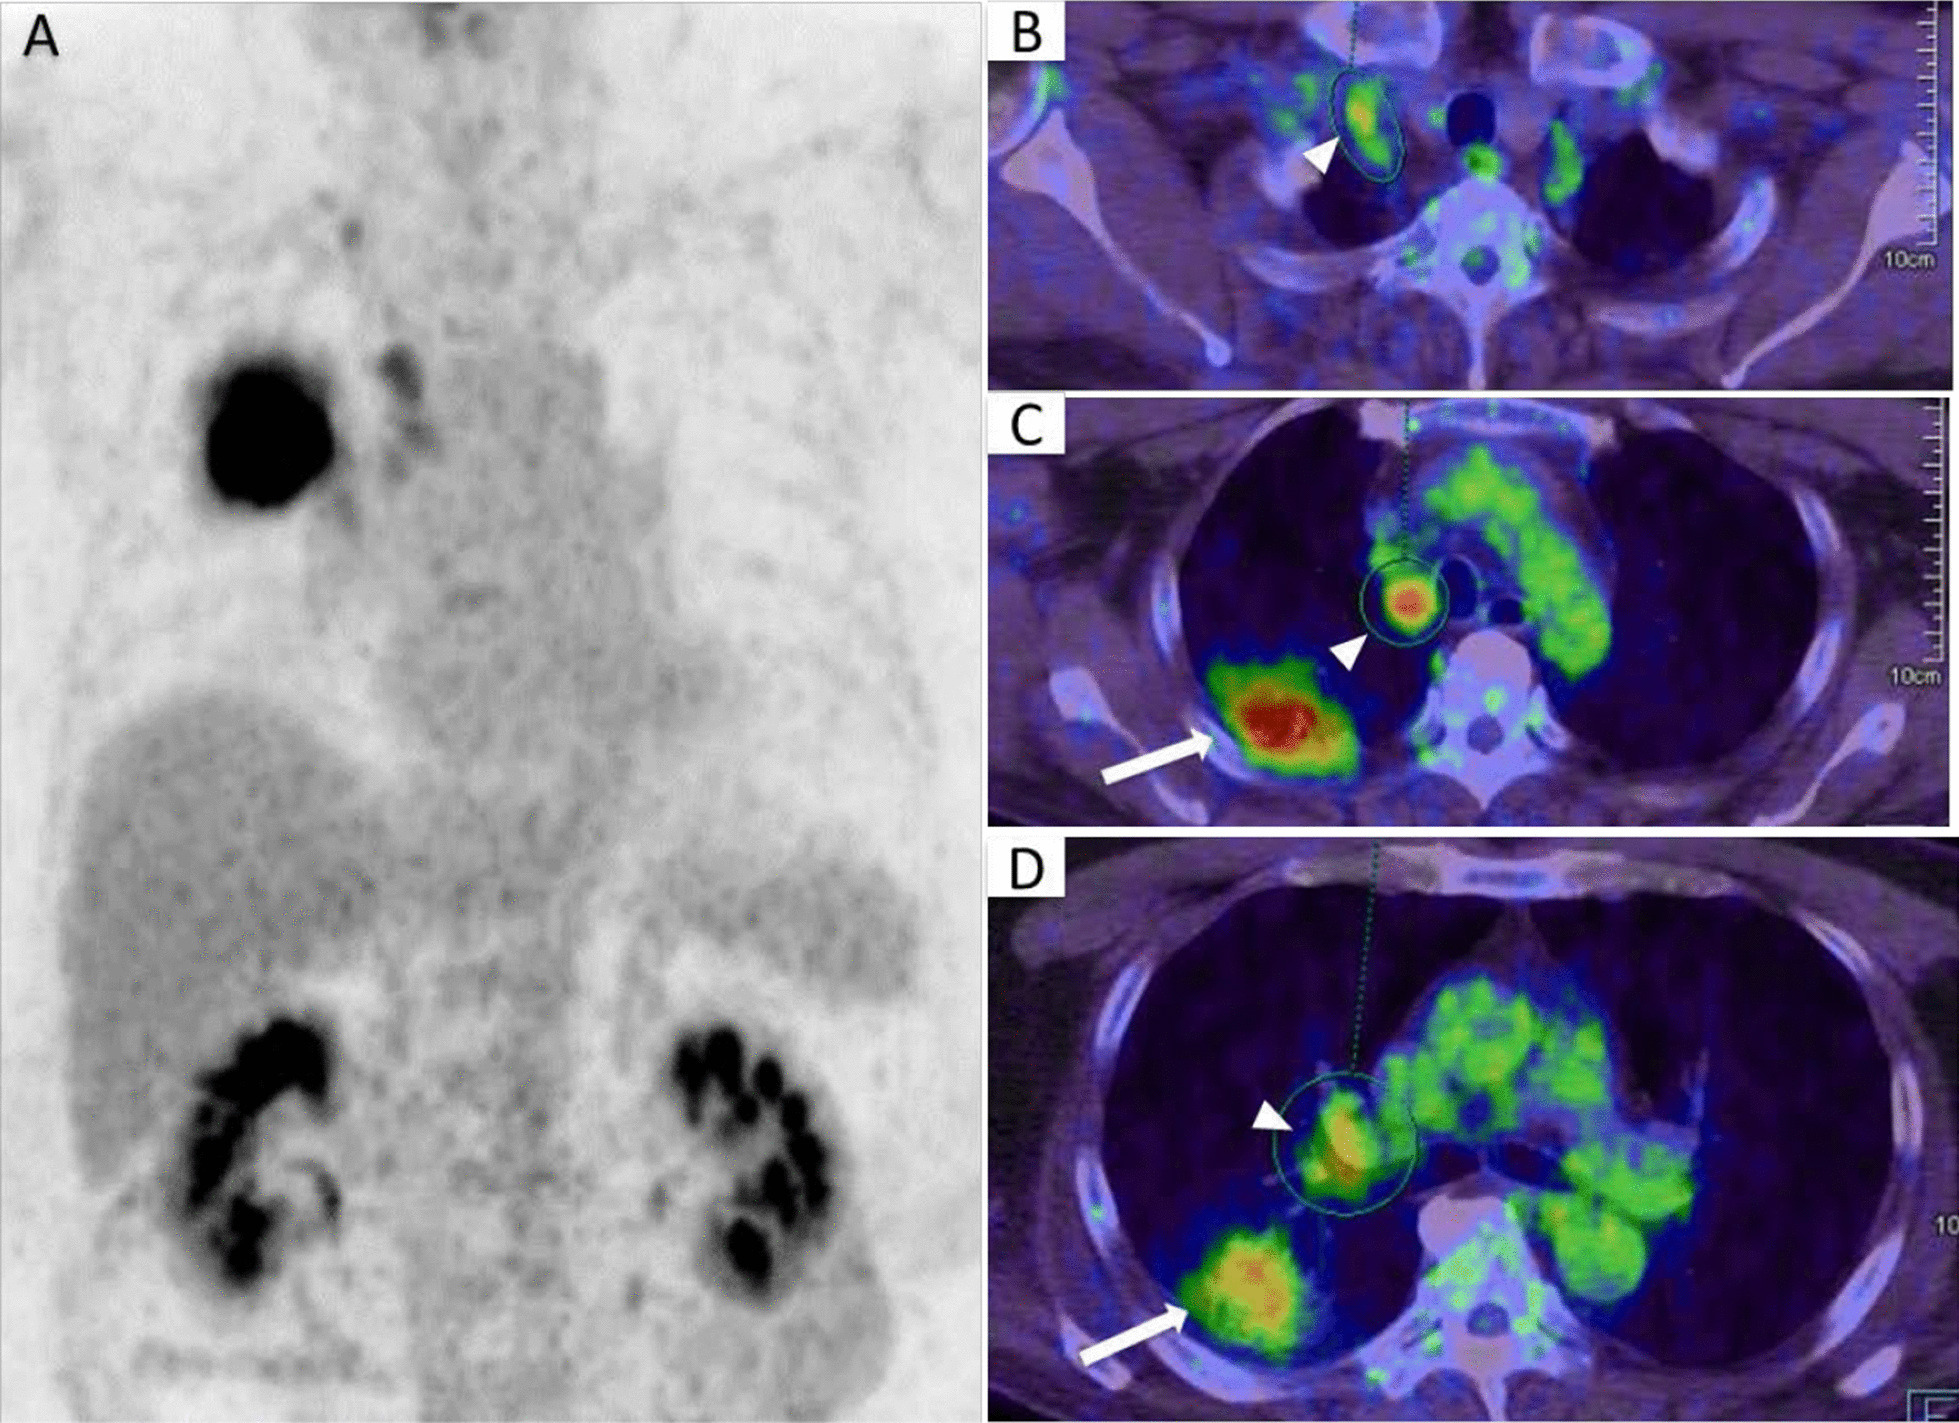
FDG-PET/CT of lung lesions. Fluorodeoxyglucose was concentrated in the primary lung lesion (arrow) and hilar, mediastinal and right supraclavicular lymph nodes (arrowheads) (A coronal view; B–D axial views)
